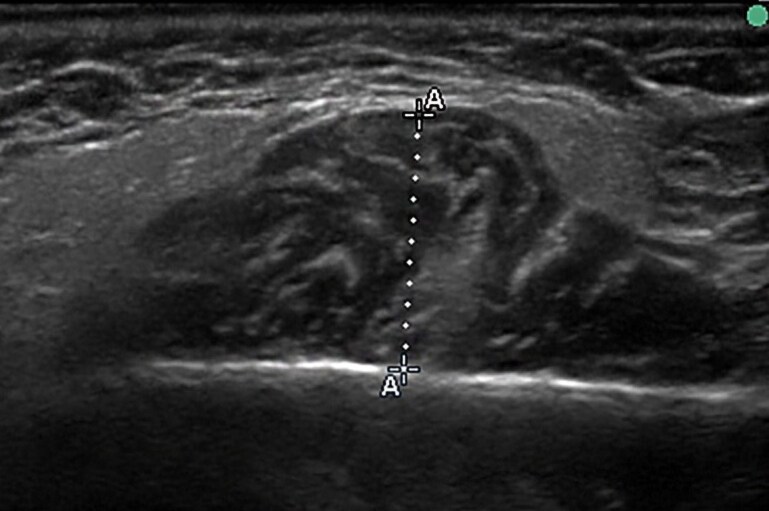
Transverse ultrasonographic image of the masseter muscle. The skin and subcutaneous tissue lie above the superficial fascia (thin echogenic line). The masseter muscle appears hypoechoic with internal striations beneath the fascia. The lateral surface of the mandibular ramus is visible as a bright echogenic contour with posterior acoustic shadowing. Electronic calipers measure the distance between the superficial fascia and the mandibular surface to assess muscle thickness, as is shown with the letter A in the figure.
